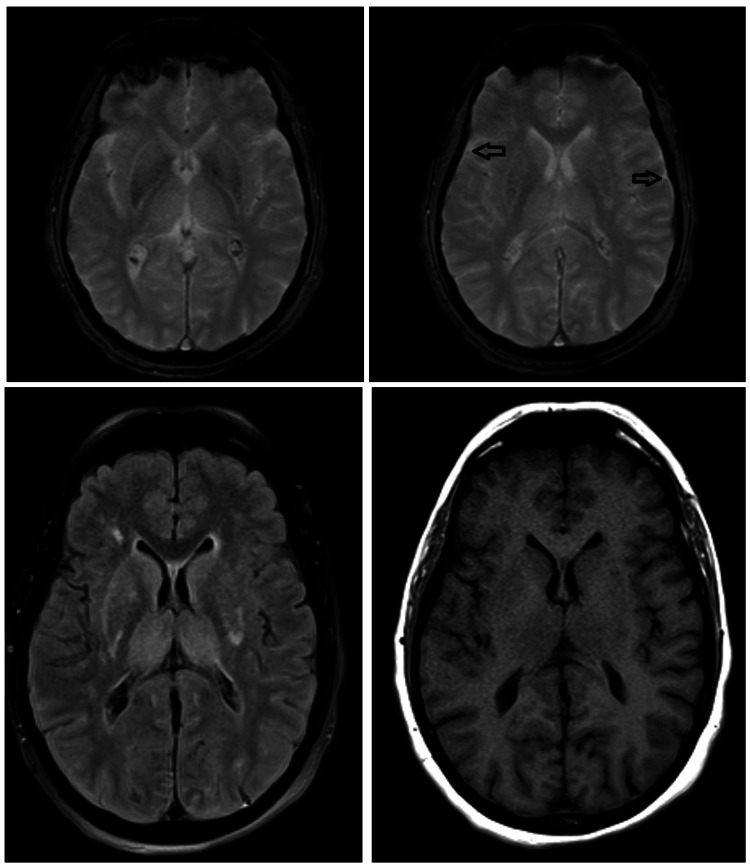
Post-operative brain magnetic resonance imaging Changes consistent with a pseudohypoxic pattern are described in the context of spinal surgical intervention. There was a diffuse thickening and pachymeningeal enhancement (arrows); these findings are compatible with a context of cerebrospinal fluid hypotension.
